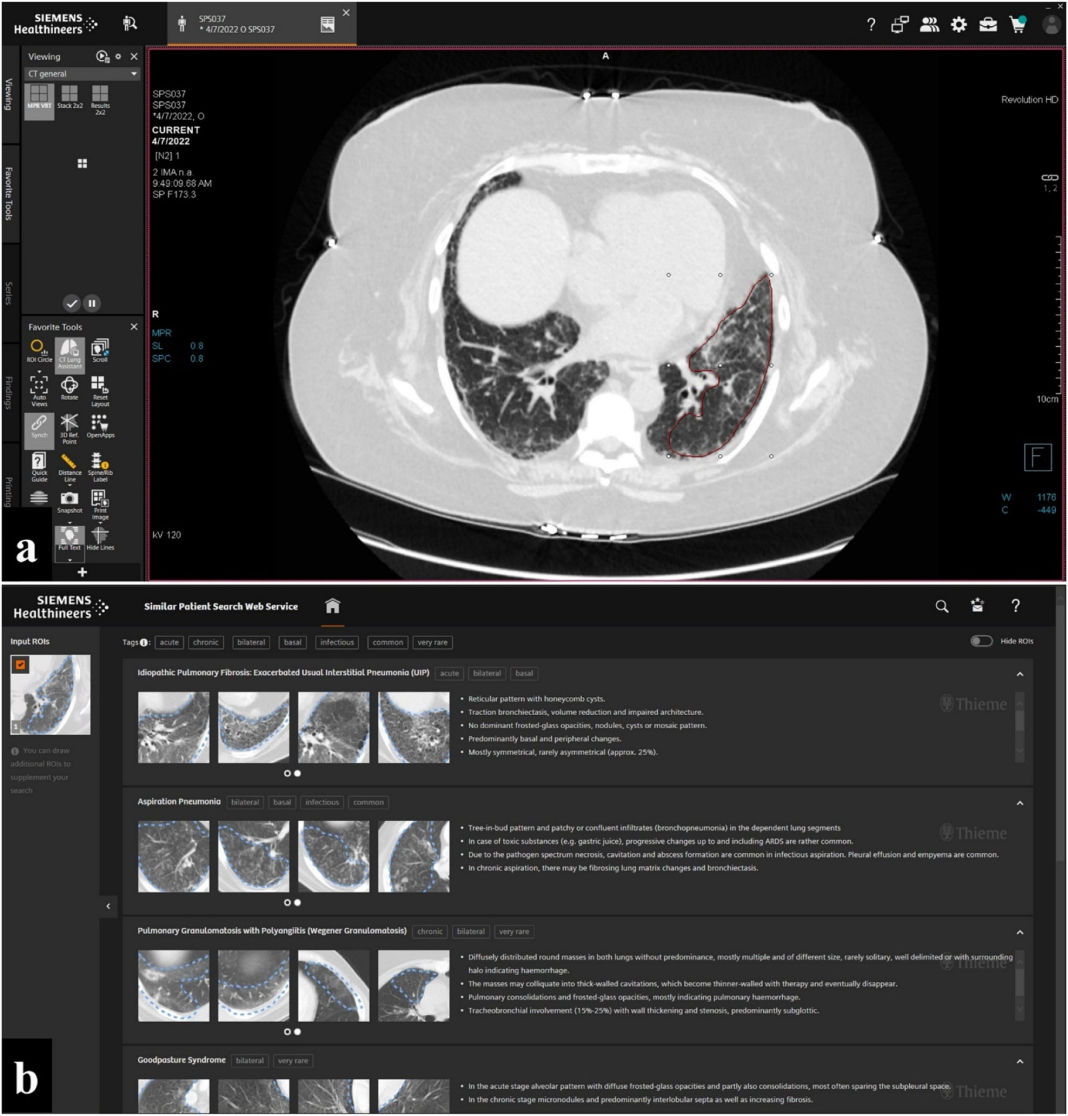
User interface of the content-based image retrieval application (Similar Patient Search Web Service (Siemens Healthineers). a Example of an input ROI on an axial high-resolution chest computed tomography in lung window settings. b List of retrieved cases with assigned pathologies starting with the most similar at the top. ROI, Region-of-interest
